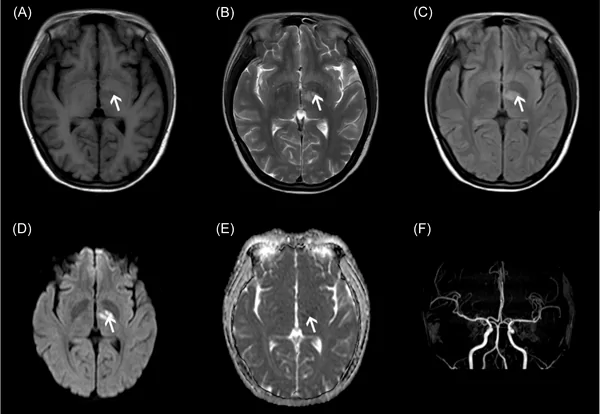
In the left basal ganglia region, small patchy areas with slightly prolonged T1 and slightly shortened T2 signal intensities are observed (A, B, white arrows). On FLAIR imaging, this area exhibits high signal intensity with blurred margins (C, white arrows). Diffusion‐weighted imaging (DWI) shows high signal intensity within this region (D, white arrows), and the corresponding ADC map shows reduced signal (E, white arrows). No abnormalities were detected on head MRA (F). ADC, apparent diffusion coefficient; MRA, magnetic resonance angiography.
